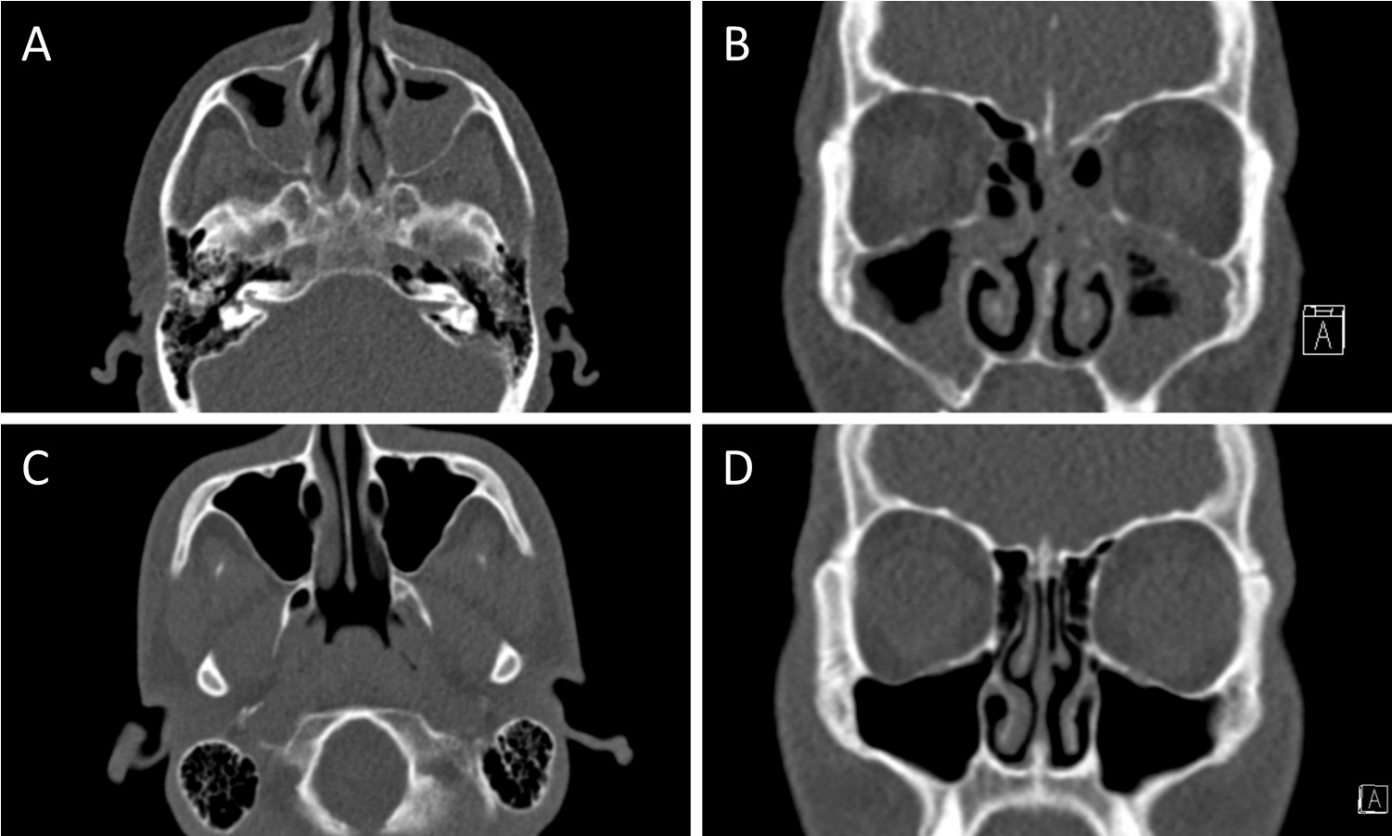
Ultra-low-dose CT-images of paranasal sinus. Axial (A) and coronal (B) reformations of 100 kV tin-filtered ultra-low-dose CT of the paranasal sinus of a 63-year-old male (DLP 2.1 mGycm; effective dose 0.012 mSv). Despite the very low dose, the diagnosis of florid sinusitis is easily established. In a 19-year-old male (axial C, coronal D), sinusitis was sufficiently excluded (DLP 1.8 mGycm; effective dose 0.010 mSv). The general image quality in both patients was rated as “moderate” by both readers.
